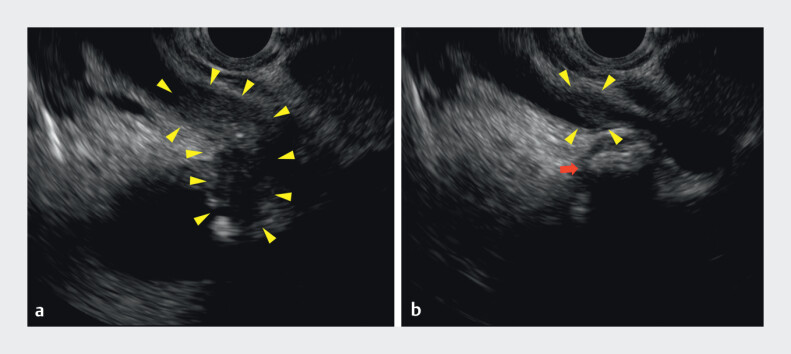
Endoscopic ultrasonography.
